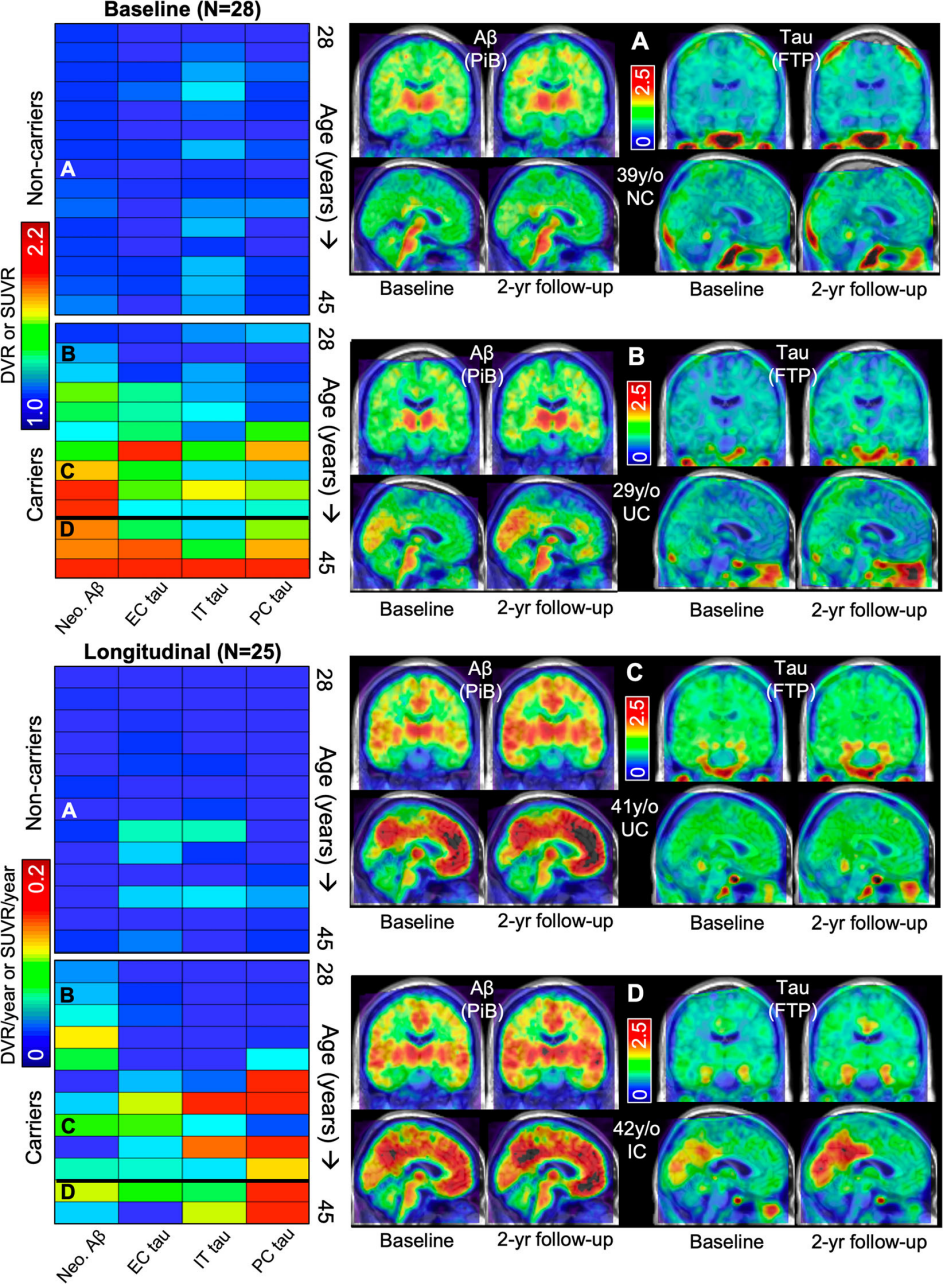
Longitudinal Aβ and tau PET images in *PSEN1* E280A carriers. *Top left*: Matrix showing baseline Aβ (PiB DVR) and tau (FTP SUVR) PET measures (color bar) for all subjects: Each column is a PET variable, and each row is a subject, separated by *PSEN1* E280A carrier status and ordered by age increasing from top to bottom (right labels; expected age at symptom onset in carriers = 44 years, 95% CI [43–45]). *Bottom left*: Matrix showing annualized Aβ and tau PET change rates (color bar) for all subjects, arranged as above. Horizontal thick black line separates cognitively unimpaired carriers (UC, above line) from cognitively impaired carriers (IC, below line). We assessed Aβ burden in a large neocortical aggregate (Neo.) and tau burden in three primary ROIs: entorhinal cortex (EC), inferior temporal gyrus (IT), and precuneus (PC). Four exemplary cases are labeled (A–D), with corresponding baseline and 2-year follow-up PET slice data shown at right. A–D: Aβ (PiB DVR, left) and tau (FTP SUVR. right) PET images at baseline and 2-year follow-up in coronal (upper) and sagittal (lower) slices for four exemplary cases, labeled in color matrices at left. Age and carrier status are given for each participant (y/o, years old; NC, non-carrier; UC, unimpaired carrier; IC, impaired carrier)
